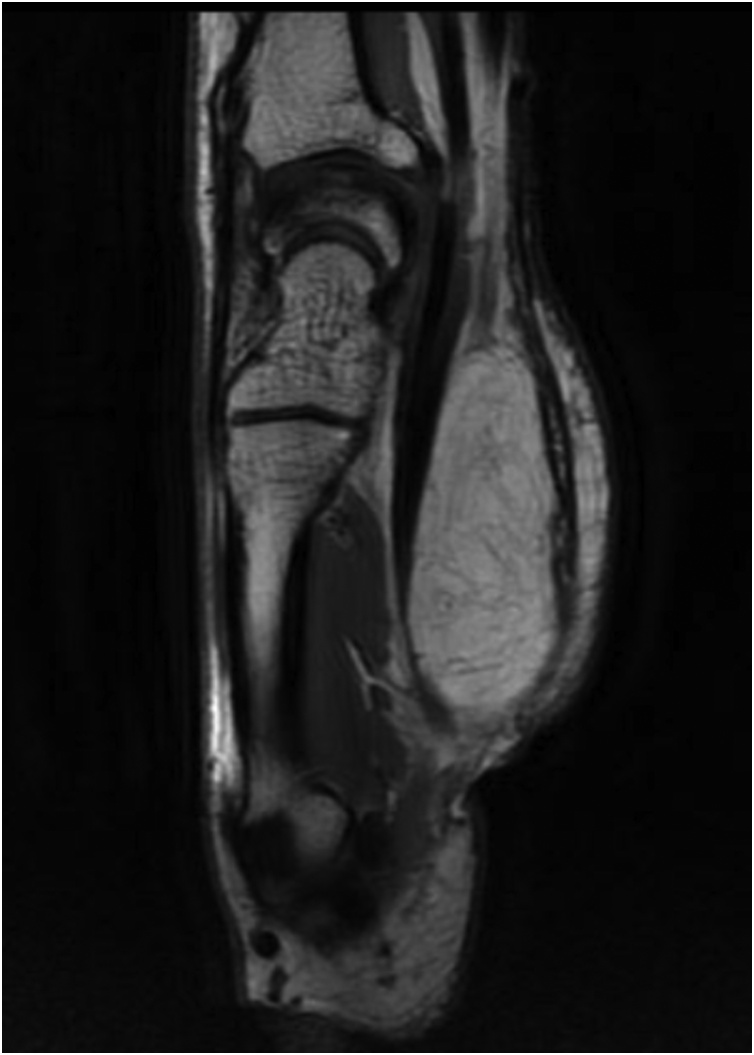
T1-weigthed sagittal MRI image showing a capsulated adipose tumour shaping the flexor tendons, with signs of invasion.
